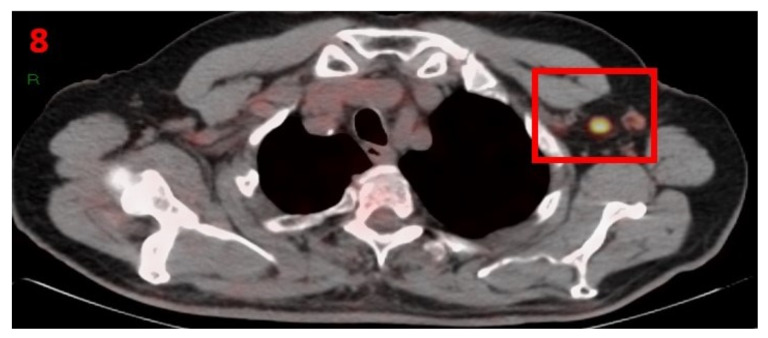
[18F]PSMA PET/CT showing hypermetabolic uptake in the 8th patient with lymphadenopathy in the left axillary region (red square).
